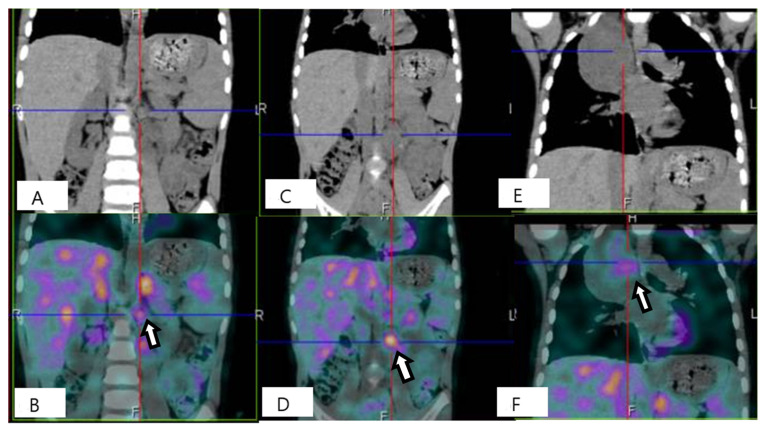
Reevaluation with ^123^I-MIBG scintigraphy confirmed the presence of uptake at both abdominal (A–D) and thoracic level without metastatic lesions (E–F).
